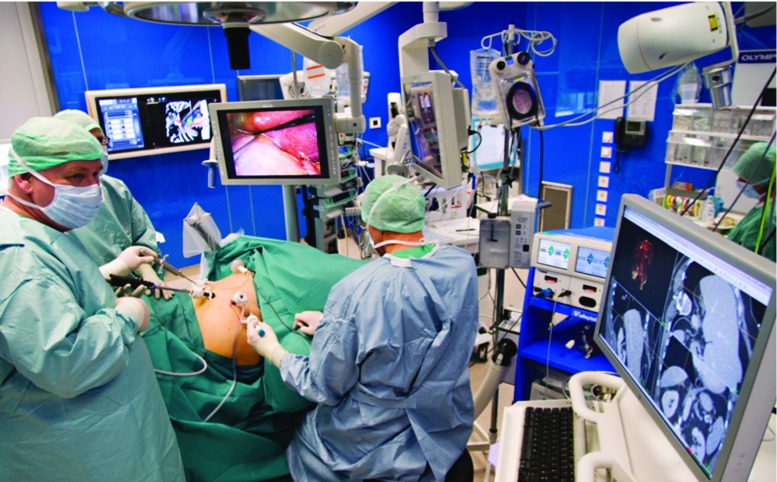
Navigation in laparoscopic surgery based on preoperative CT images
